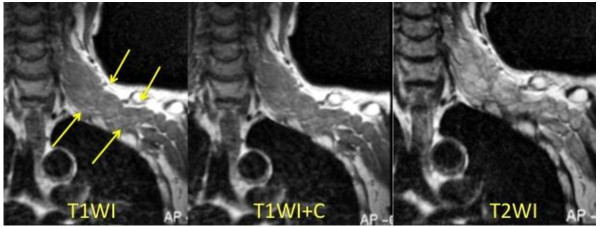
MRI images. Magnetic resonance imaging images. Magnetic resonance imaging showed a continuous, multinodular, plexiform tumor from the left C5 to C7 nerve root along the course of the brachial plexus to the left brachia. The tumor showed the same intensity as the muscle on T1-weighted images (T1WI), and slightly higher intensity on T2-weighted images (T2WI). Gadolinium-enhanced images (T1WI+C) showed no enhancement of the tumor. There was no sign of the tumor inside the vertebral canal.
